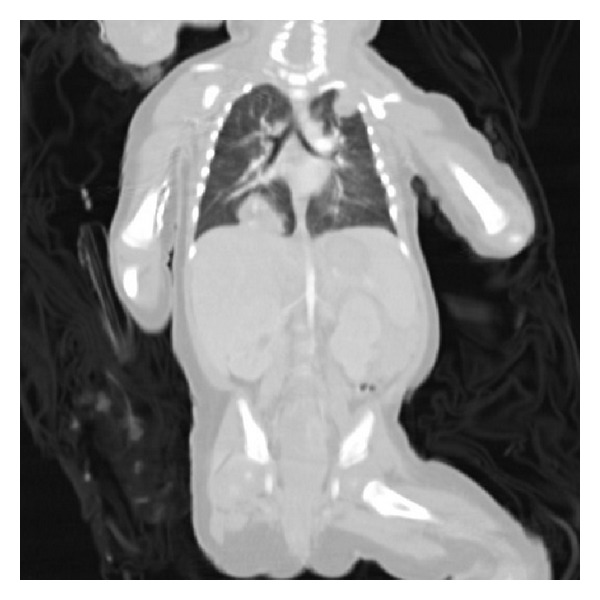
CT of the chest with metastasis.
